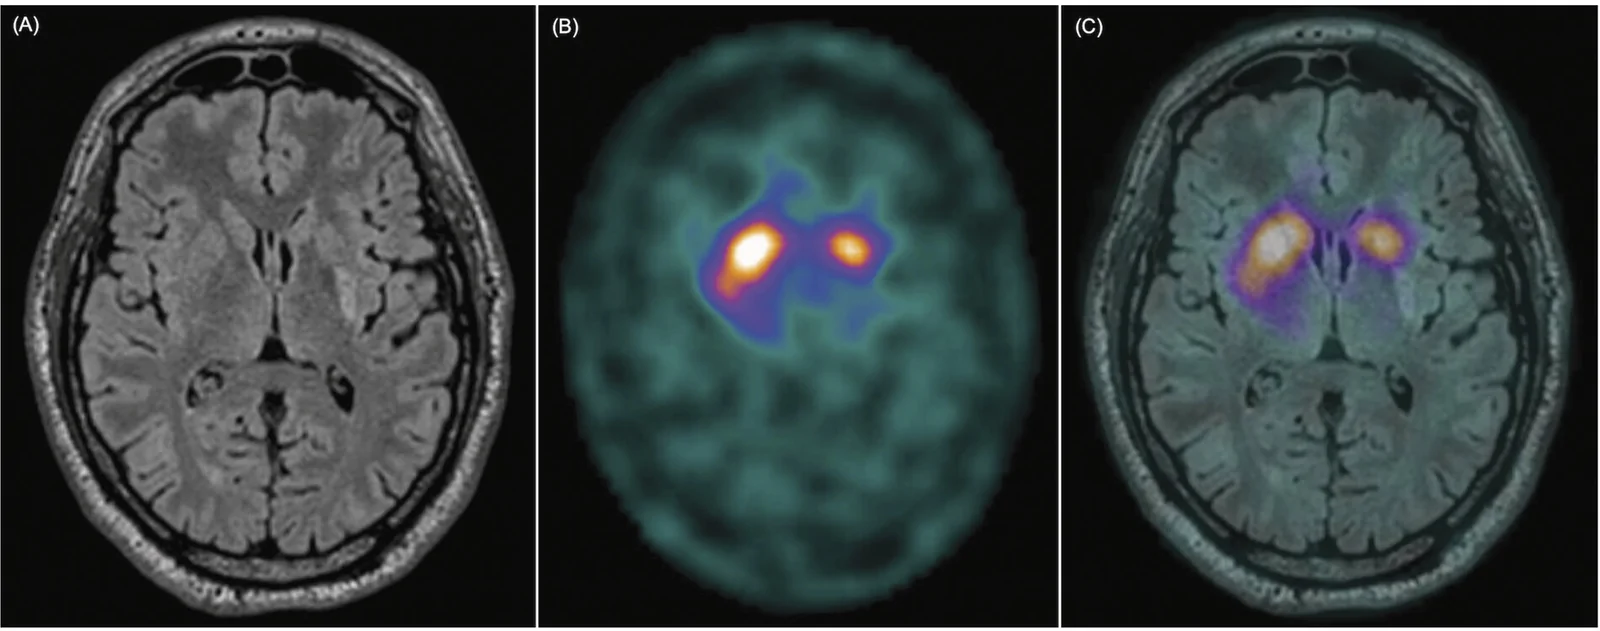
Post-stroke parkinsonism. MRI and [
